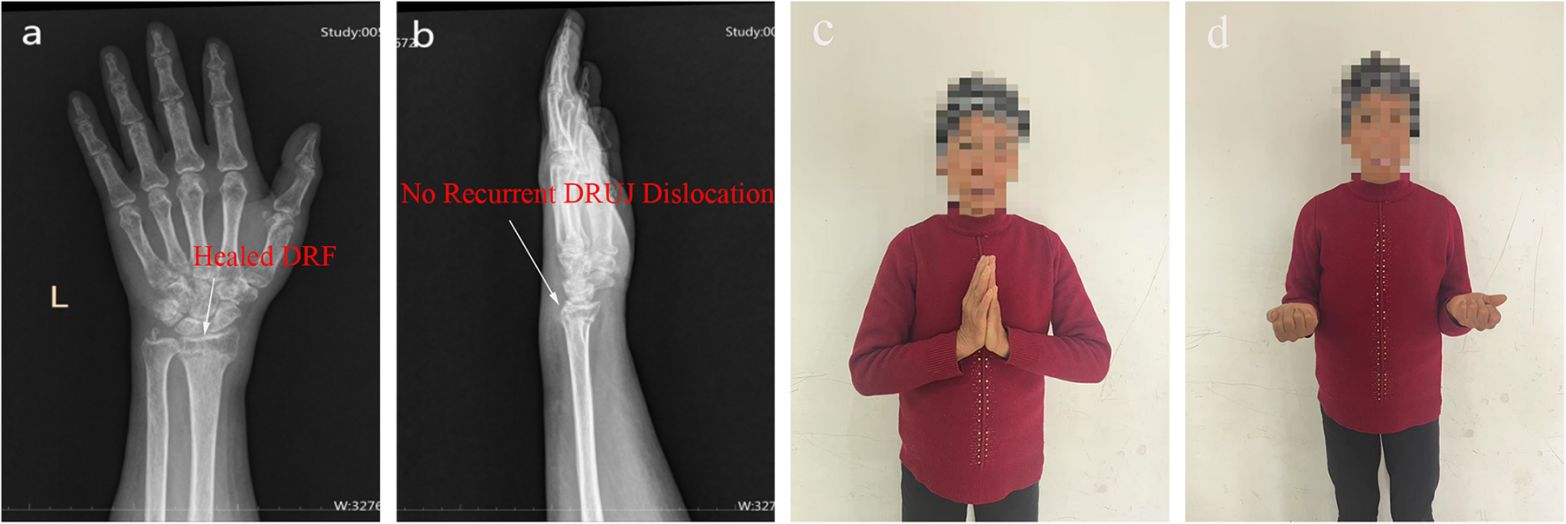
Two-month follow-up DRs after fixation. (a,b) satisfactory healing of the radius, with correction of the distal radioulnar joint dislocation. (c,d) the patient demonstrates good range of motion in wrist rotation and dorsiflexion. DR, digital radiograph.
